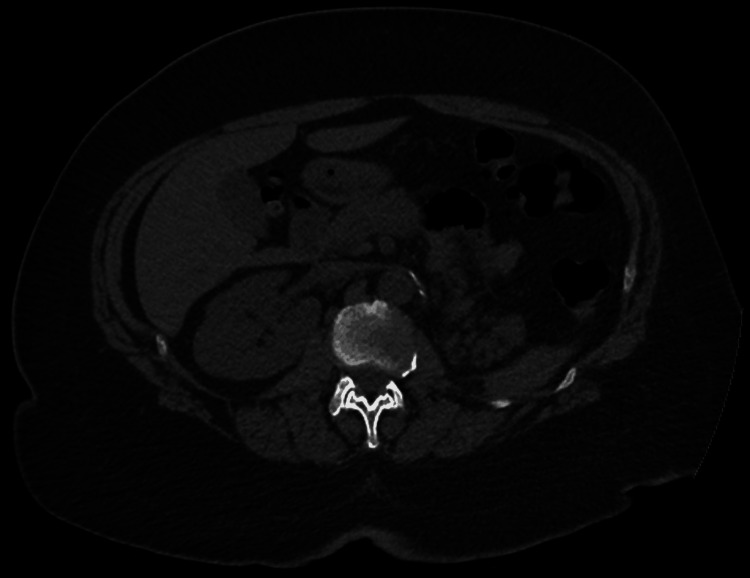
CT abdomen on admission showing a solitary right kidney and a surgically absent left kidney.
